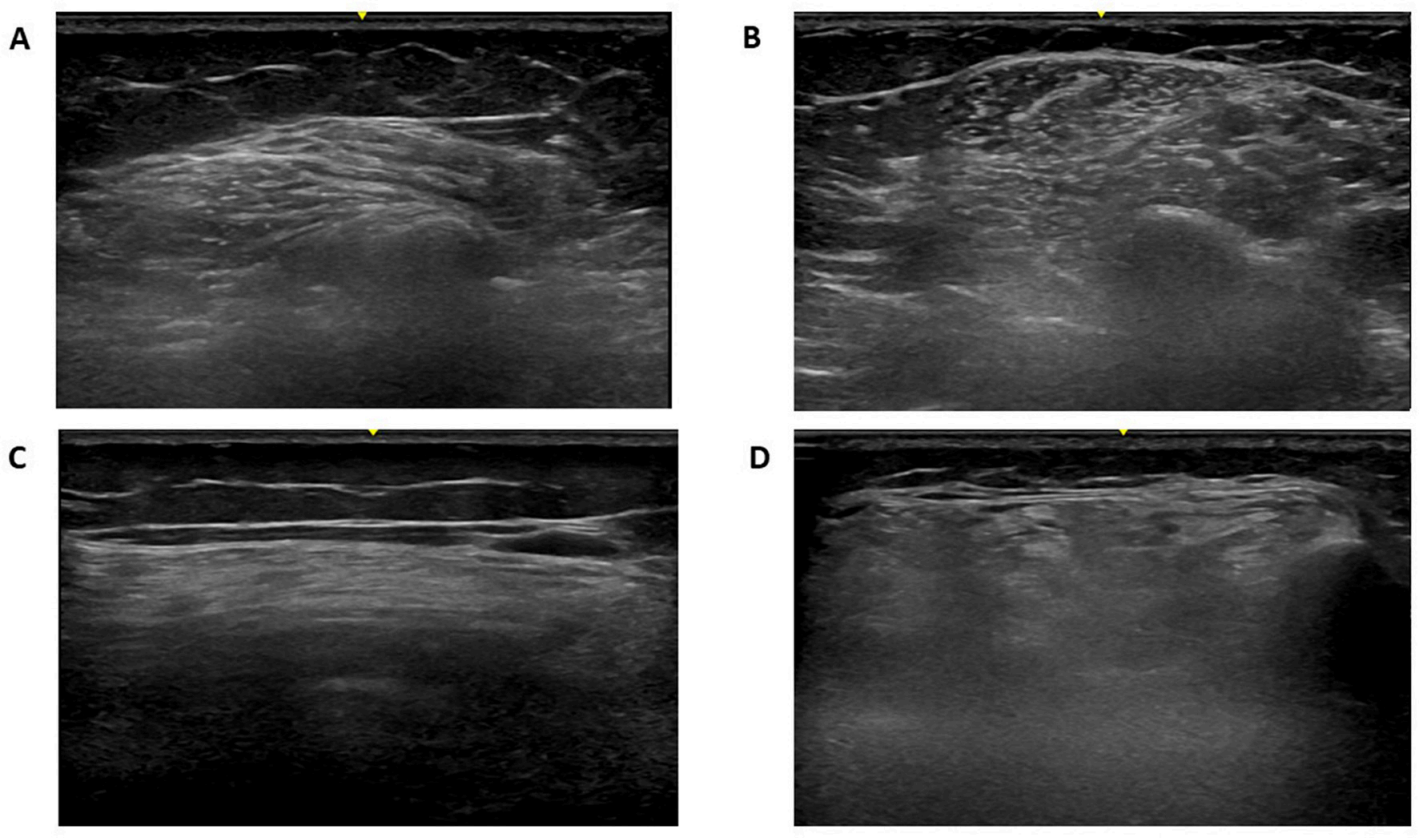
Muscle ultrasound of patient 5 with GFPT1-related congenital myasthenic syndrome demonstrating severe myopathic changes. Muscle ultrasound images from Patient 5 with GFPT1-related congenital myasthenic syndrome, demonstrating advanced myopathic changes graded by the modified Heckmatt scale. (A) Right deltoid (Grade 3) (B) Right biceps brachii (Grade 3); (C) Right vastus lateralis (Grade 4) (D) Right tibialis anterior (Grade 4).
